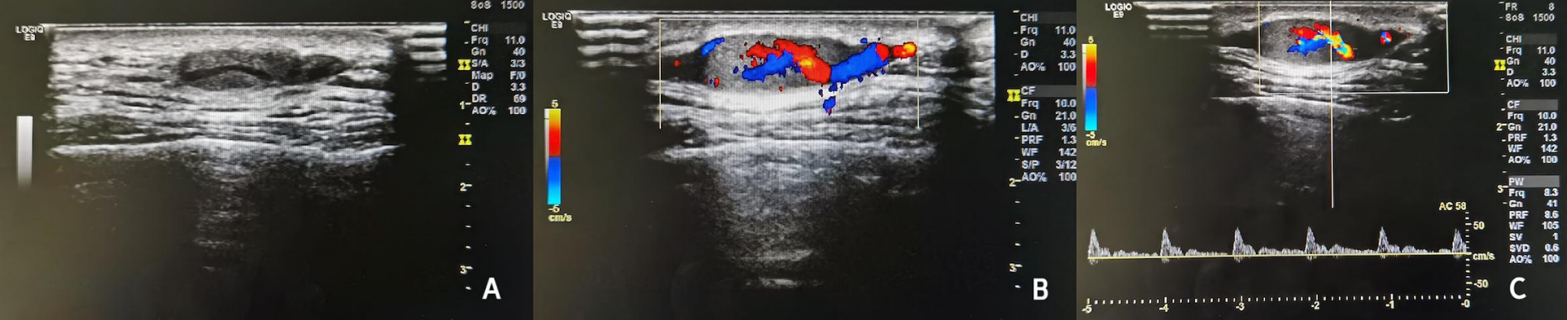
Ultrasound images. (A) The B-mode image showed a well-defined, hypoechoic oval lesion within which a tubular anechoic structure was observed extending from bottom to top. (B) The Color Doppler imaging exhibited pulsatile color flow signals originating from the superficial temporal artery. (C) The Spectral Doppler demonstrated continuous arterial blood flow.
